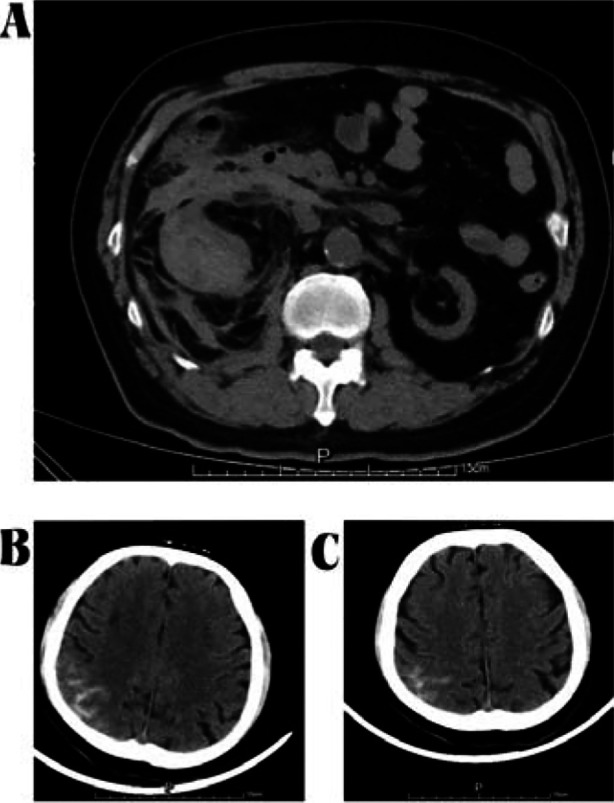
Computed tomographyscan of the abdomen showed abnormal morphology, disappeared renal portal structure, strip slightly high density shadow, surrounding multiple flocculous high density and unclear boundary in the right kidney and indicated him renal rupture (A) Computed tomographyscan of the skull showed high-density shadow in the top part of the right brain groove and indicated him subarachnoid hemorrhage at admission (B) and Three days after admission (C).
